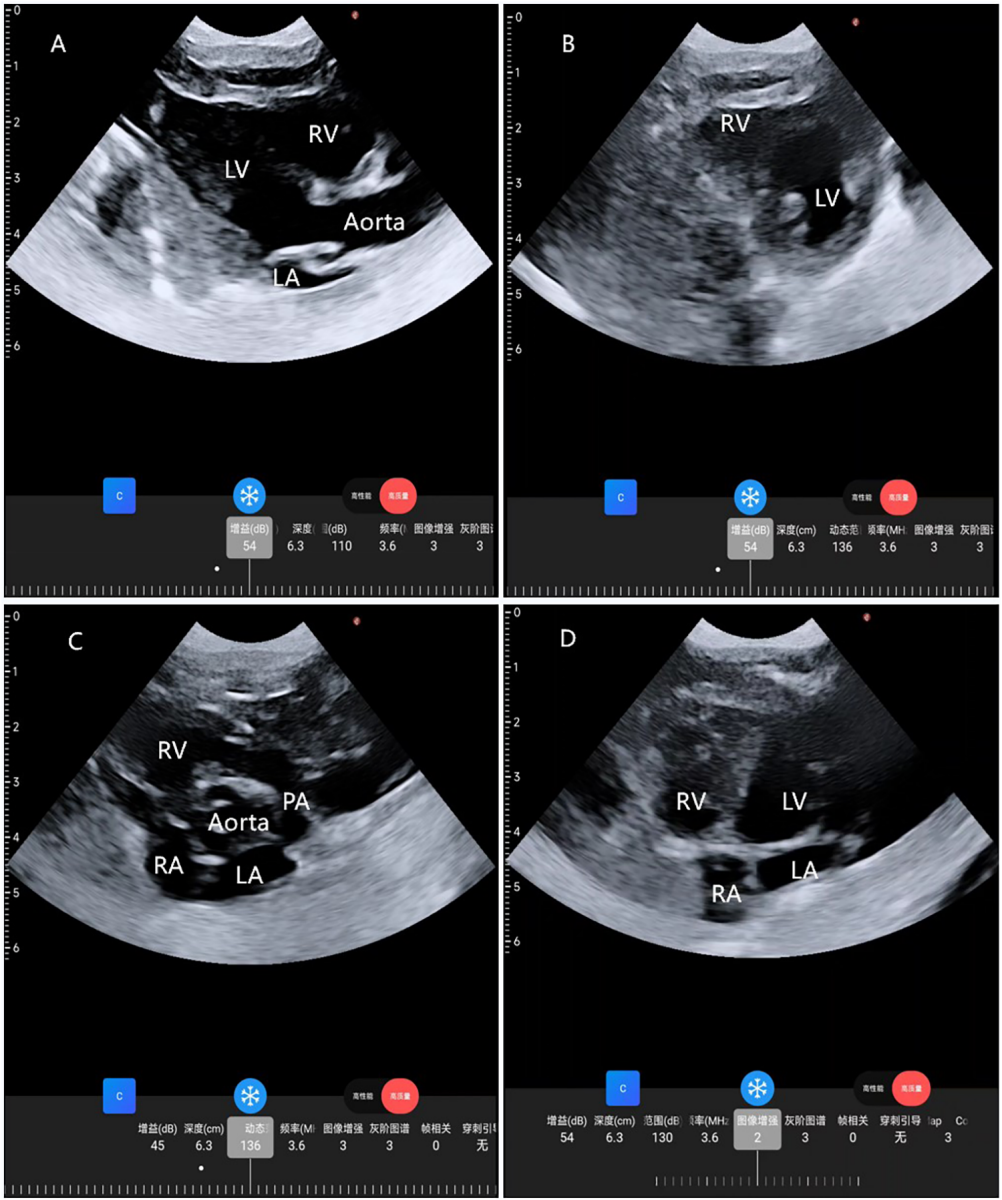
Cardiac images from four standard views taken by a wearable cardiac ultrasound monitoring imager. (A) Parasternal long-axis view; (B) parasternal LV short-axis view; (C) parasternal aortic short-axis view; (D) apical 4-chamber view. RA, right atrium; RV, right ventricle; LA, left atrium; LV, left ventricle; PA, pulmonary artery.
